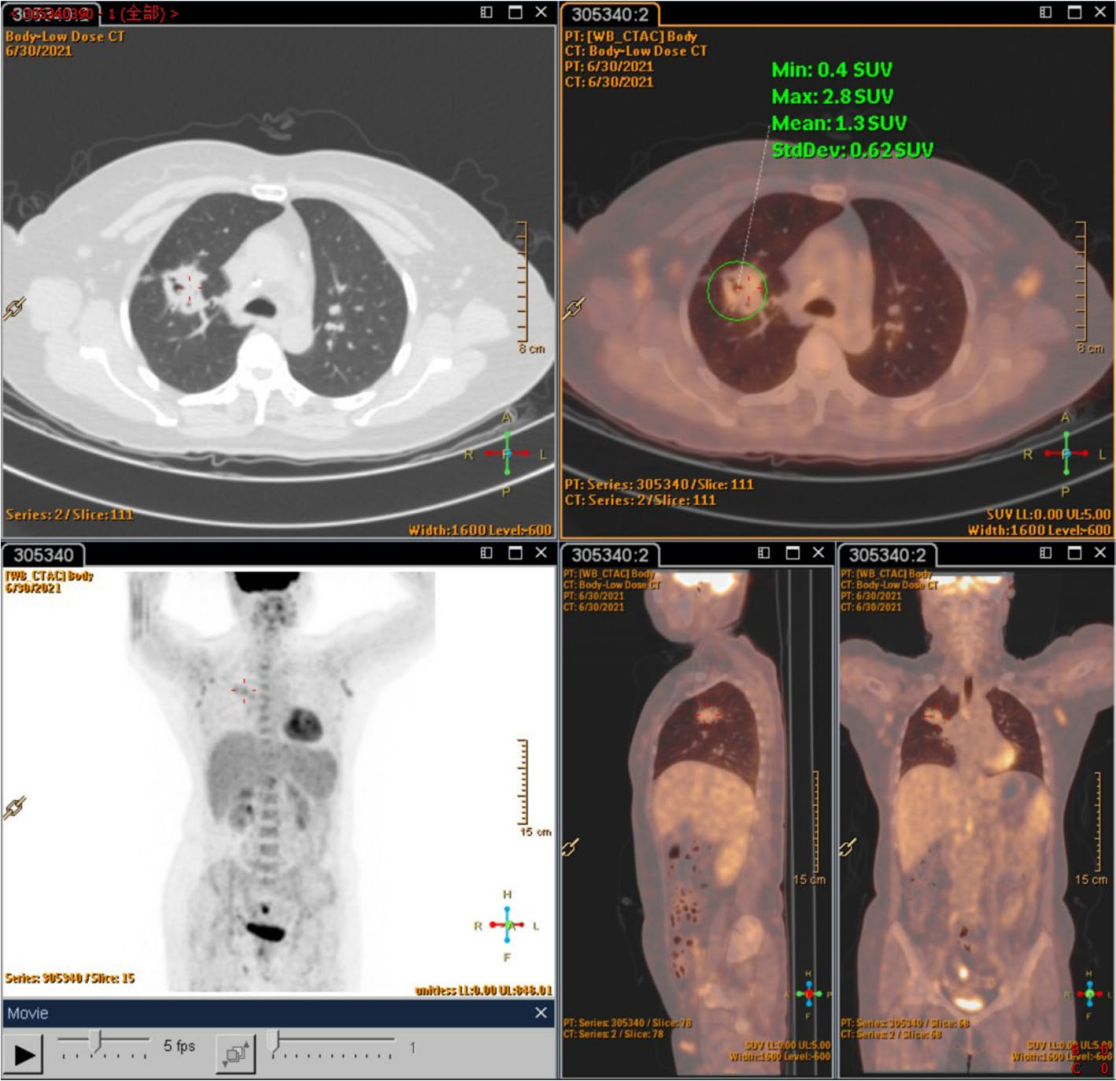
PET-CT. June 30th, 2021 PET-CT indicated space-occupying lesions in the upper lobe of the right lung with increased metabolic activity. Peripheral lung cancer was considered
